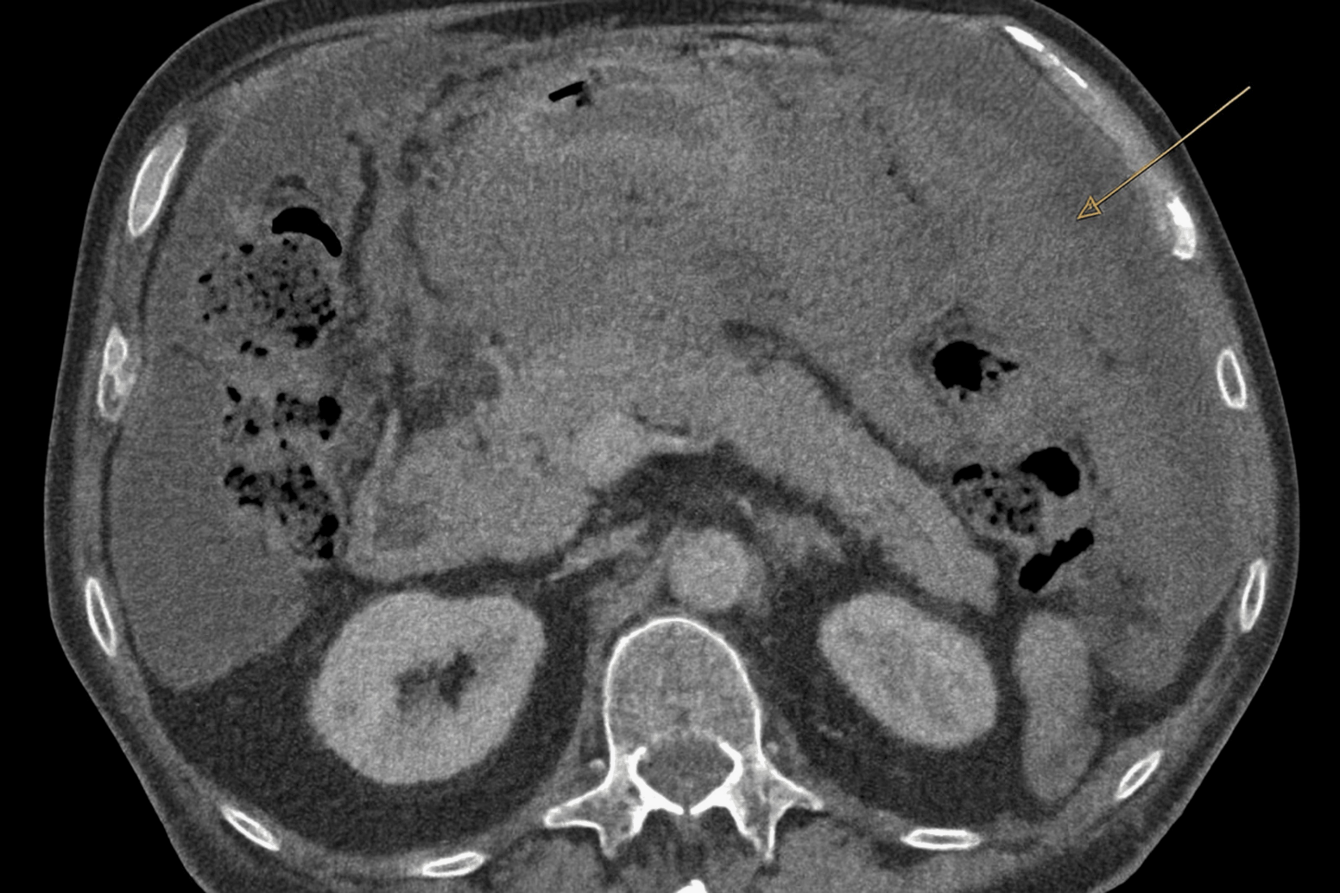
Axial computed tomography (CT) of the abdomen (portal venous phase) showing omental thickening and ascites. Axial computed tomography image of the abdomen (portal venous phase) demonstrates a sheet of omental thickening in the left hypochondriac region (arrow) with associated moderate ascites.
